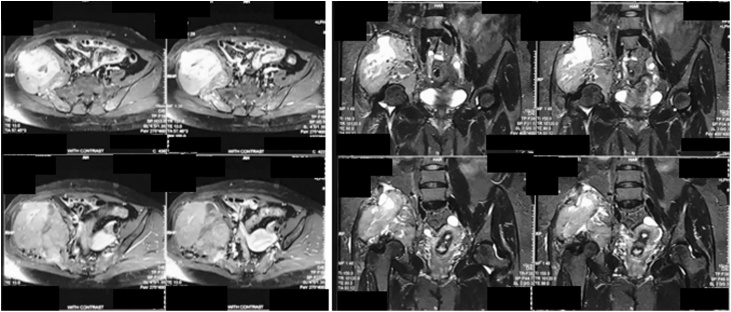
MRI Examination of the Pelvis.
